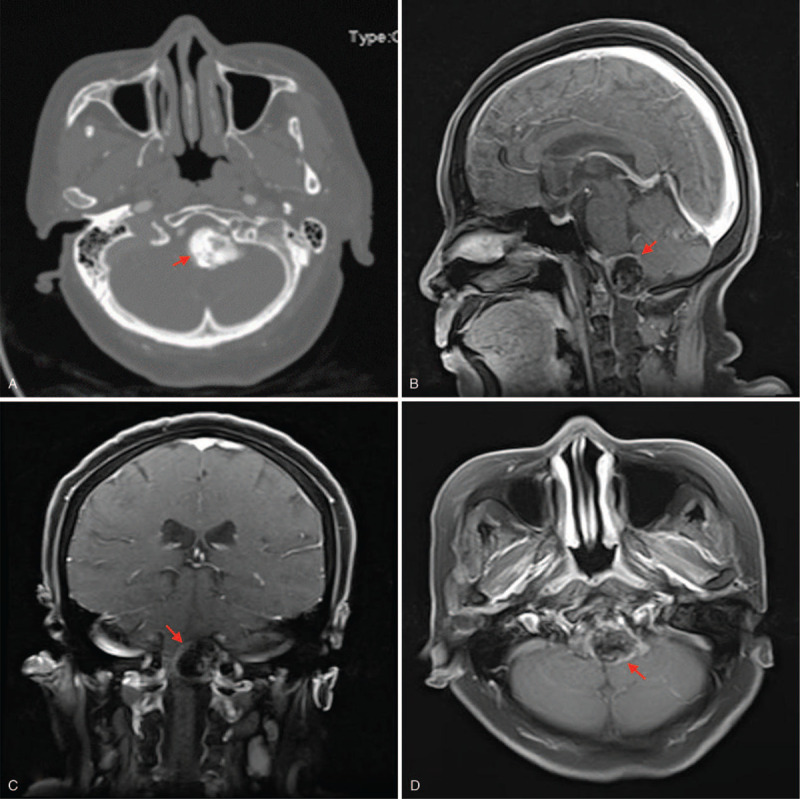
A-D are preoperative CT and MRIs. A: Is a CT scan showing a calcified mass at occipital cistern. B-D: Are MRIs showing an irregular lesion in the posterior part of the medulla oblongata with enhanced edges signifying calcifications. *Red arrow = tumor*. CT = computed tomography, MRI = magnetic resonance imaging.
